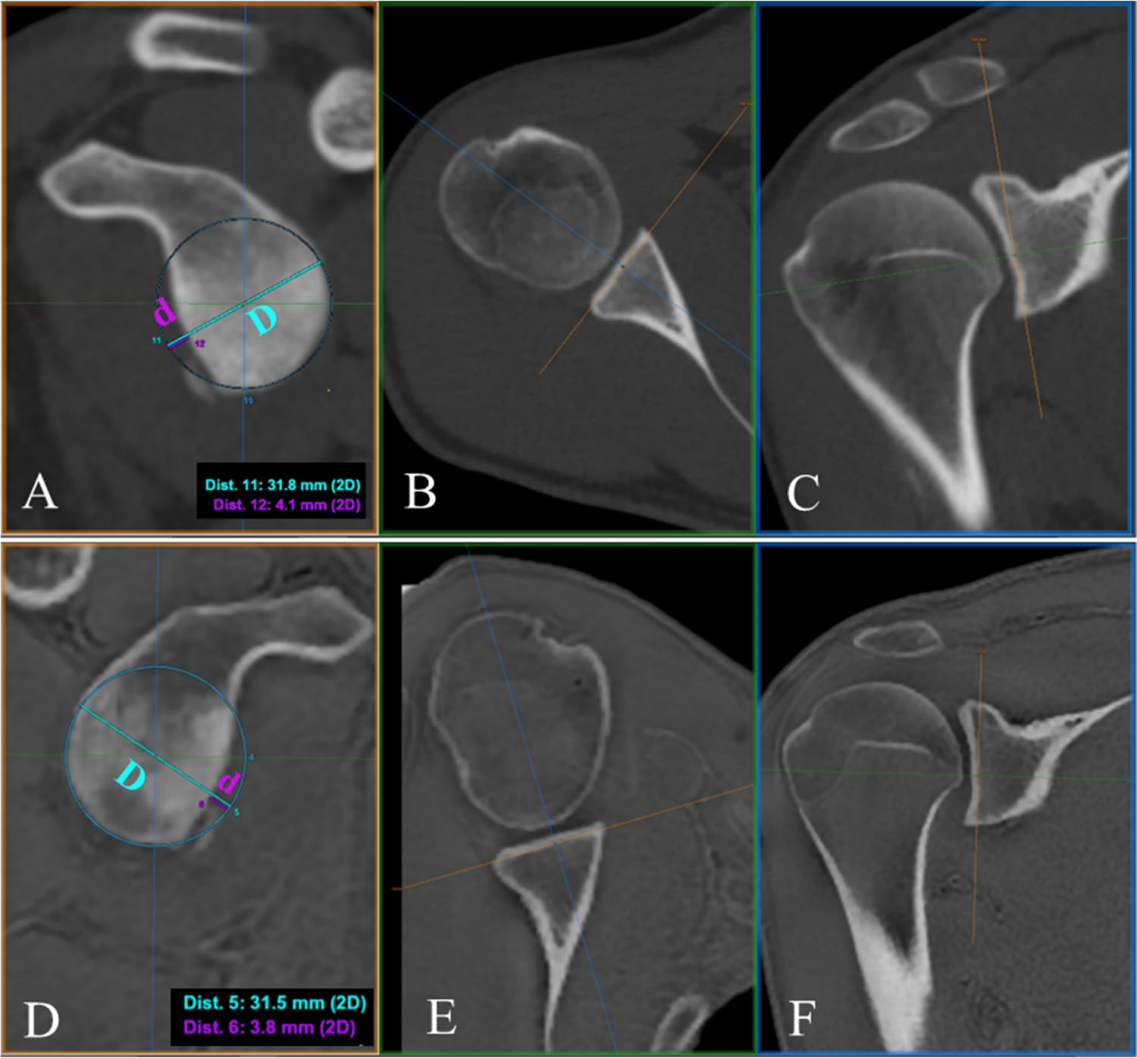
GT measurement. En face sagittal MPR view of the glenoid showing the best-fit circle placement on CT (A) and ZTE (D) images from the same patient, with proper angulation on their corresponding axial (B, E) and coronal (C, F) planes
